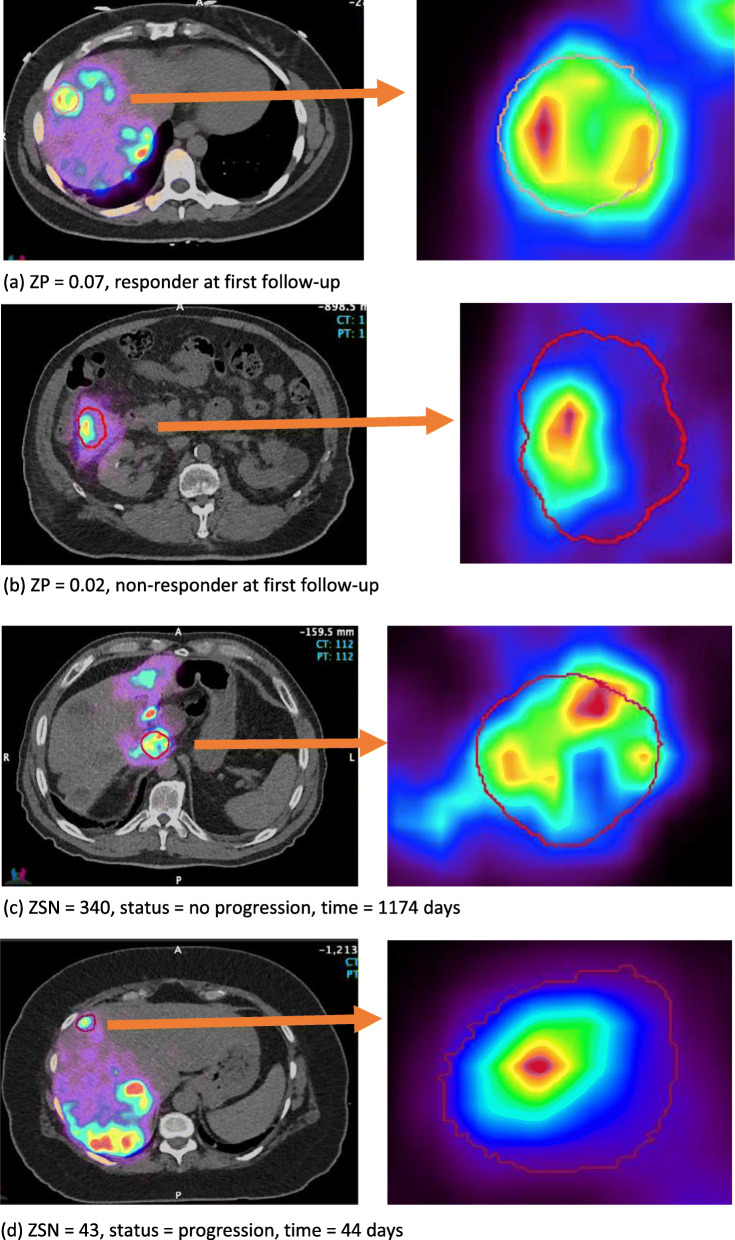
Example ^90^Y PET/CT images with CT-defined lesion contours (left: PET/CT axial slice showing the anatomical position within liver, right: magnified lesion on PET). a Lesion with large ZP value corresponding to responder. b Lesion with small ZP value corresponding to non-responder. c Lesion with large ZSN value corresponding to no progression at 1174 day. d Lesion with small ZSN value corresponding to progression in 44 days
